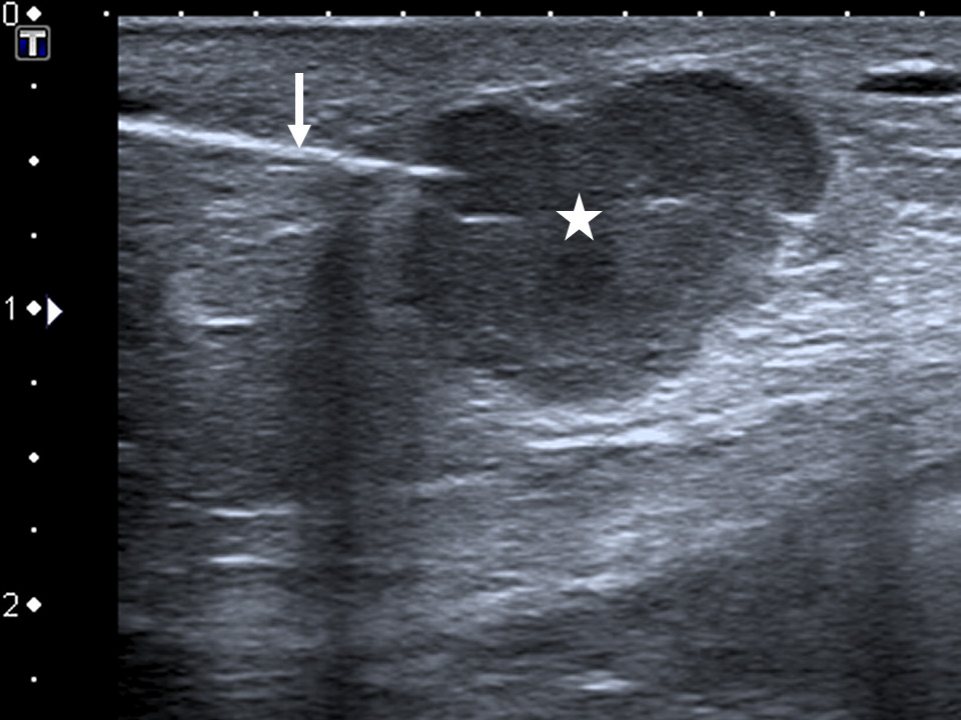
Ultrasound image of the left breast showing the microlobulated hypoechoic nodule (star) with posterior enhancement and the biopsy needle (arrow).
